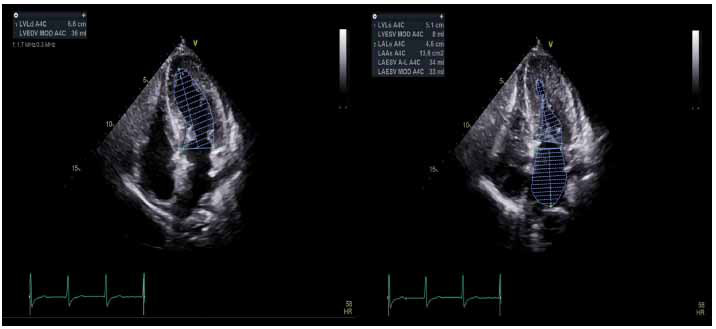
Quantitative assessment of LVEF in the patient with HOCM, demonstrating hyperdynamic systolic function with LVEF of 77%. Using the Simpsons biplane method, LVEF is obtained by averaging measurements in the apical 4-chambers (shown) and apical 2-chamber views.
